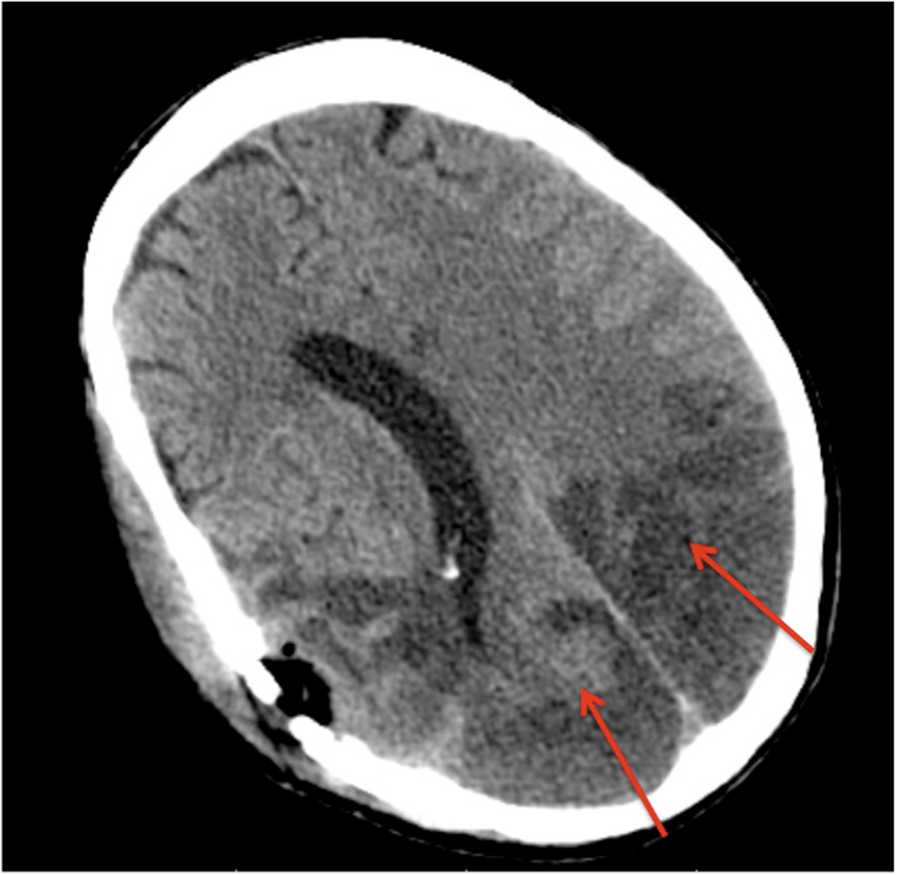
A cranial CT-scan at a later stage of disease demonstrated additional bilateral infarct lesions in the posterior circulation territory.
